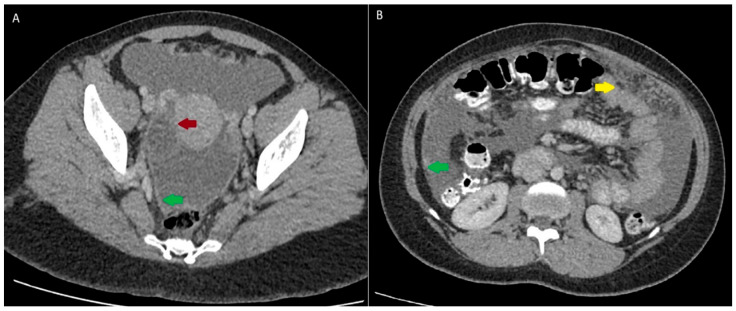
Abdominopelvic computed tomography. (A) Thin-walled hypodense right adnexal lesion (red arrow) and smooth thickening of the peritoneum (green arrow). (B) Nodular thickening of the peritoneum (green arrow) and nodular thickening of the greater omentum (yellow arrow).
